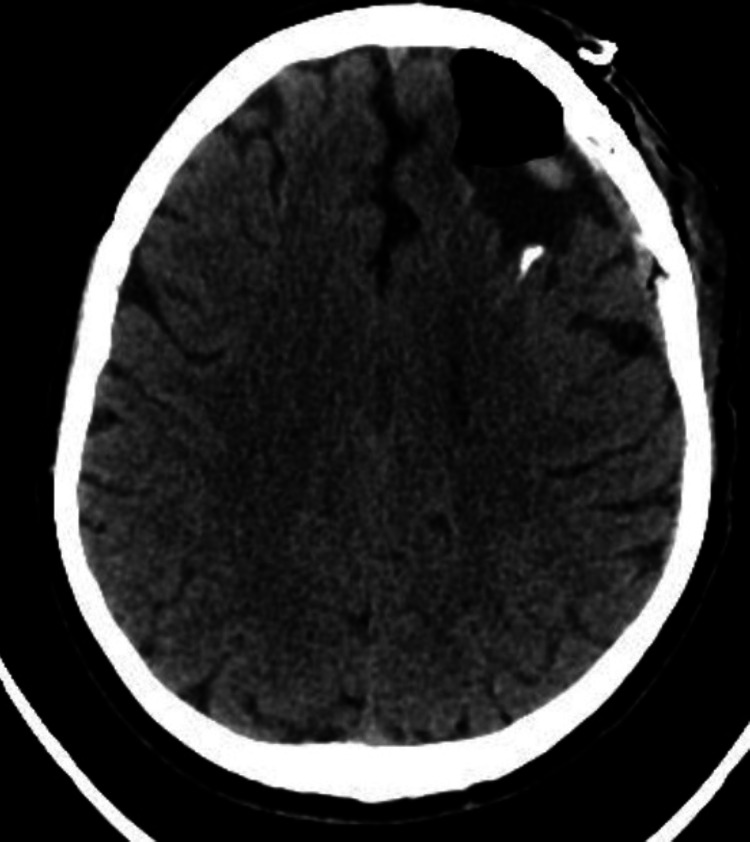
Post-surgical Computed Tomography scan, showing complete removal of the cyst - Axial view.
